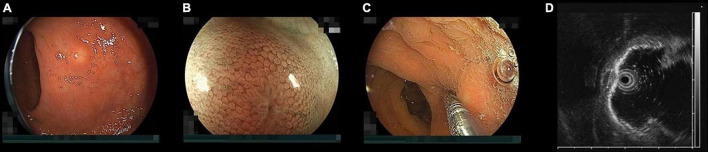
Endoscopic findings of a rectal neuroendocrine tumor (NET). (A) Typical rectal NET endoscopically looks like a yellow submucosal tumor covered with normal mucosa (B) magnified view shows “expanded normal pit pattern” because NETs originate from the deep mucosa and expansively grow toward the submucosa through the muscularis mucosa expanding the pits on the surface of the mucosa covering the NET. (C) When pushing a NET using the tip of a miniature probe, the NET moves with its mucosa but a submucosal tumor from the muscularis separately moves under the mucosa. (D) Endoscopic ultrasound at 20 MHz can correctly distinguish a NET from a submucosal tumor. The high-echoic submucosal layer can be identified between the NET and the muscularis. The NET was measured 5.2 mm in diameter with measuring function prepared to an endoscopic ultrasound unit.
